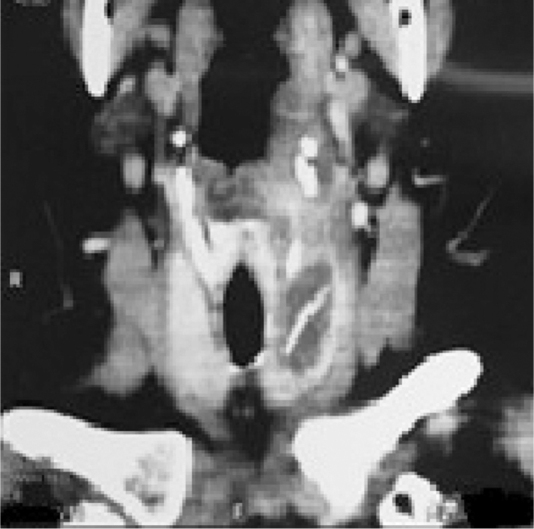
CT showed juxtaposed collection on the left thyroid lobe with a foreign body inside.
